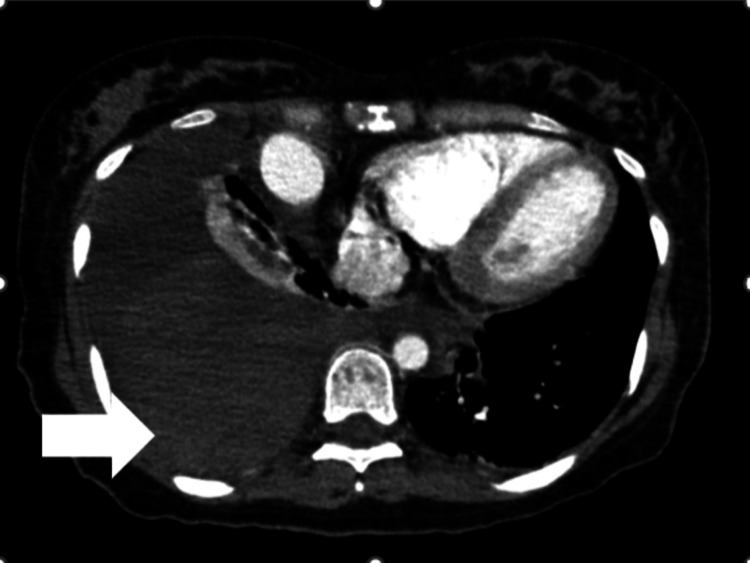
Contrast-enhanced chest computed tomography scan Contrast‑enhanced axial chest CT showing a substantial right‑sided hemothorax. High‑density coagulated blood products are seen layering dependently along the dorsal thoracic cavity (arrow).
